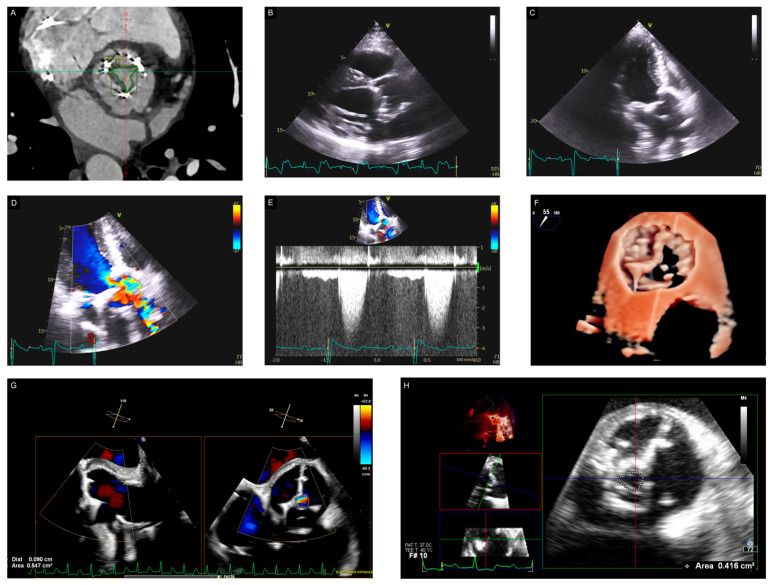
A 38-year-old male with a history of a bicuspid aortic valve underwent SAVR at the age of 30. A CT scan (Panel A) demonstrated thickening of the aortic valve prosthesis leaflets, most notably the noncoronary-facing and right coronary-facing leaflets, both of which are hypomobile. Significant thickening extends to the base of the right and noncoronary cusps at the valve plane. A TTE examination showed that the aortic valve prosthesis leaflets were calcified with reduced opening from parasternal and apical views (Panels B,C). The systolic mean Doppler gradient was 28 mmHg (EOA 1.4 cm^2^) (Panels D,E). A TEE confirmed the severity of the stenosis (Panels F–H); all the leaflets had markedly reduced opening. They were calcified except for the leaflet towards the left coronary cusp, which was not well visualized. By planimetry, the aortic valve area was between 0.4 and 0.5 cm^2^, indicative of severe aortic stenosis.
